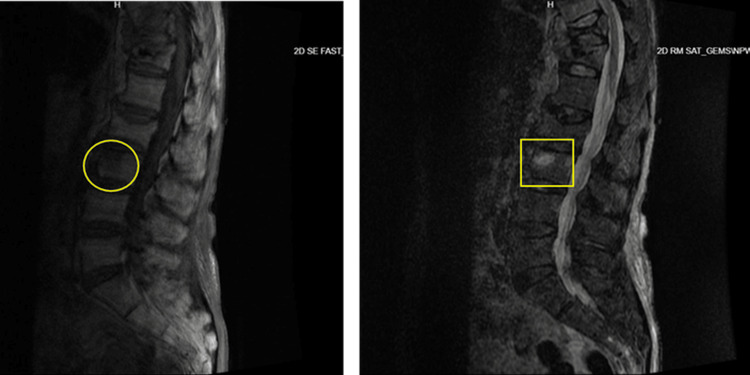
MRI of the lumbar spine without contrast: T1-weighted (left) and STIR (right) images. Yellow marks indicate a compression fracture at L3. STIR, short tau inversion recovery
